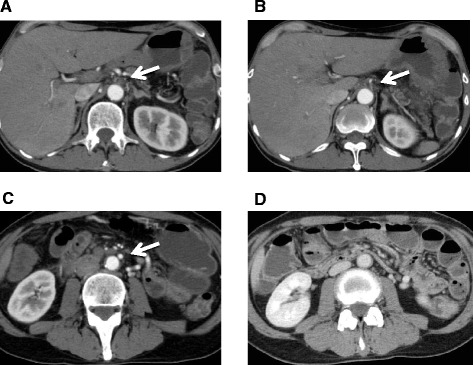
Contrast-enhanced computed tomography findings at the time of admission. The orifices of the celiac (a) and superior mesenteric arteries (b) are occluded. The inferior mesenteric artery is patent and slightly dilated (c). Contrast enhancement of the bowel wall seems normal (d)
